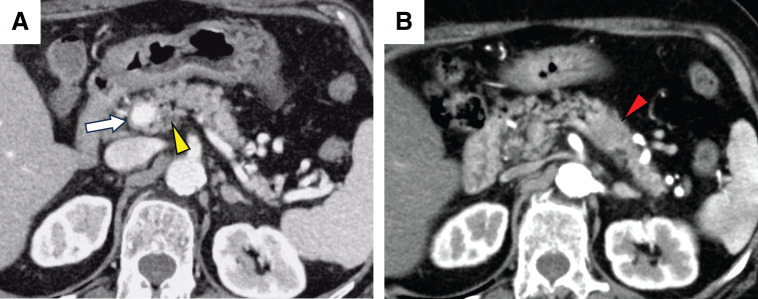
CT images. (A)–(B) are arranged in craniocaudal order. (A) The ventral pancreas (yellow arrowhead) encircles the PV (white arrow), consistent with a PAP. (B) A hypovascular tumor was observed at the pancreatic body–tail junction (red arrowhead). PAP, portal annular pancreas; PV, portal vein
